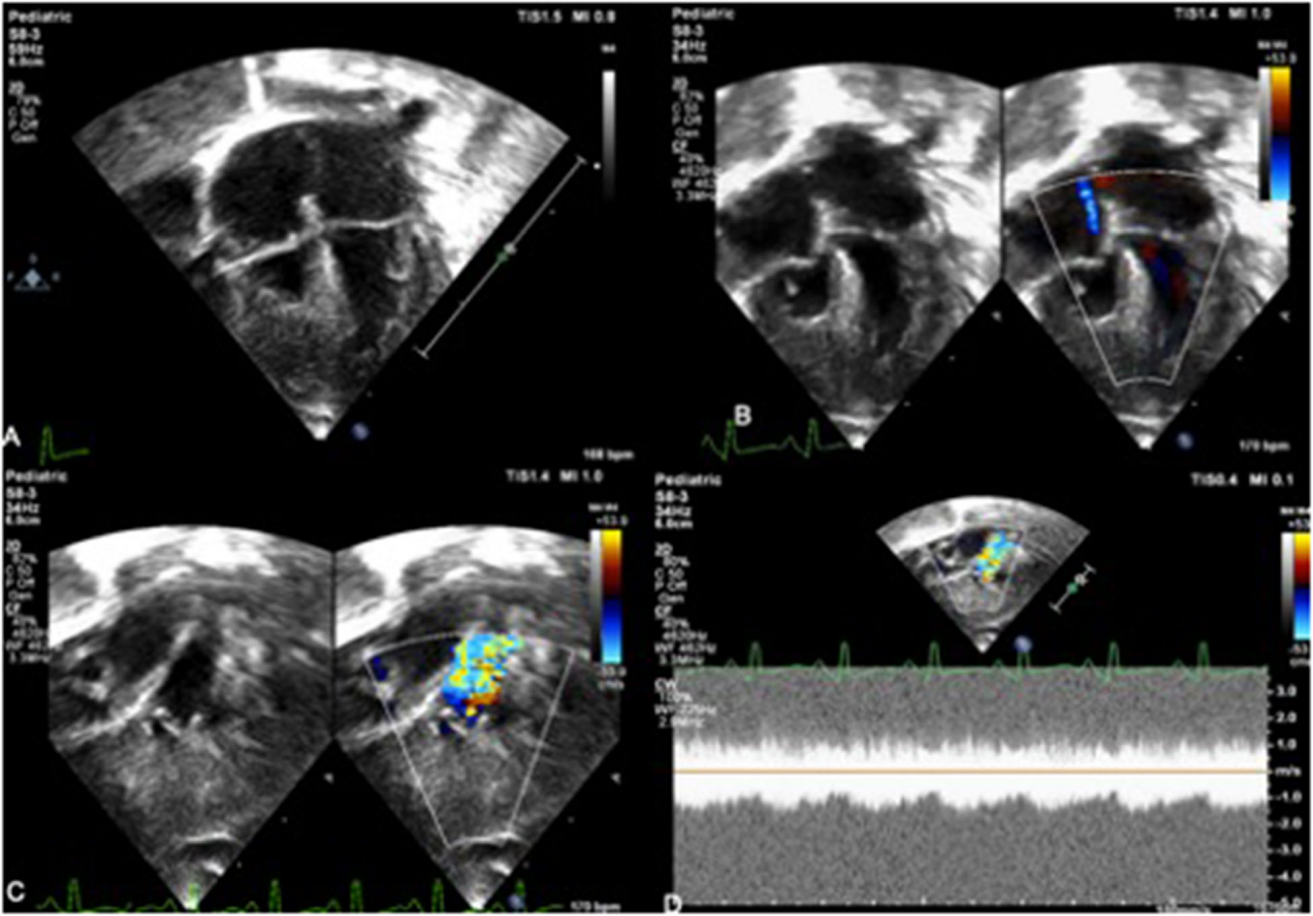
Echocardiogram showing hypoplastic and hypertrophied right ventricle with TV *Z* score-1.95. b Color doppler showing tricuspid regurgitation jet (RVSP 110 + mean RAP). b, c PDA seen filling the MPA. d CW showing the continuous flow across MPA due to its filling from PDA
